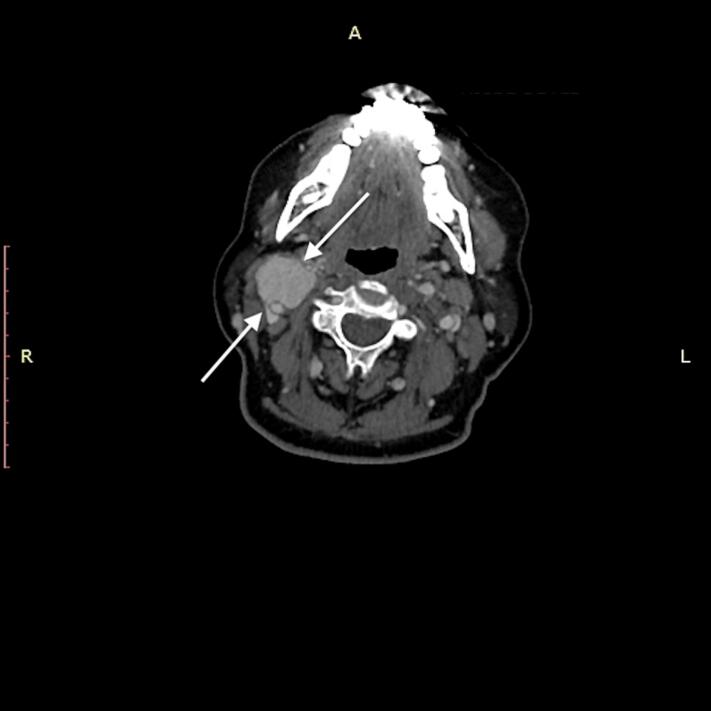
Computed tomography image of the carotid body tumor.
